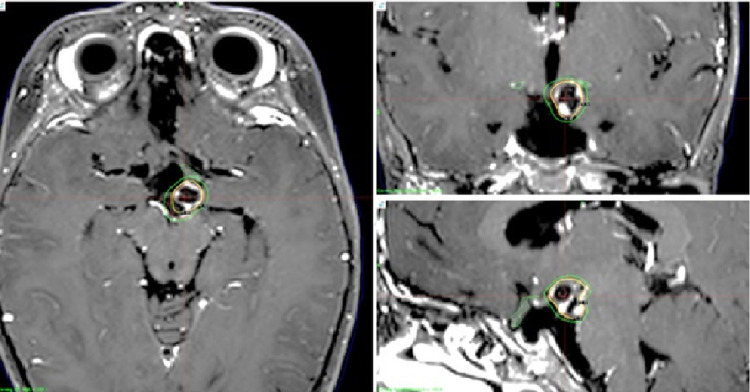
Case 2 Magnetic resonance images of a two-year-old girl with recurrent craniopharyngioma. A small left suprasellar lesion was treated with the prescribed dose of 14 Gy at the tumor margin. Irradiation took 32.8 minutes.
